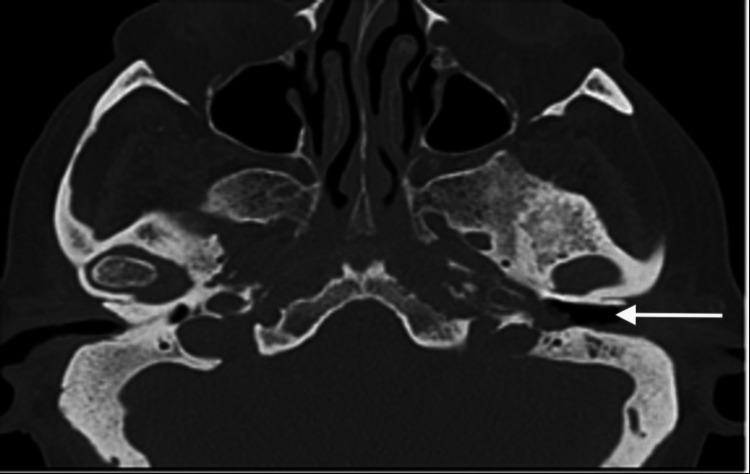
Plain CT head showing left skull base osteomyelitis, a characteristic finding in necrotizing otitis externa
